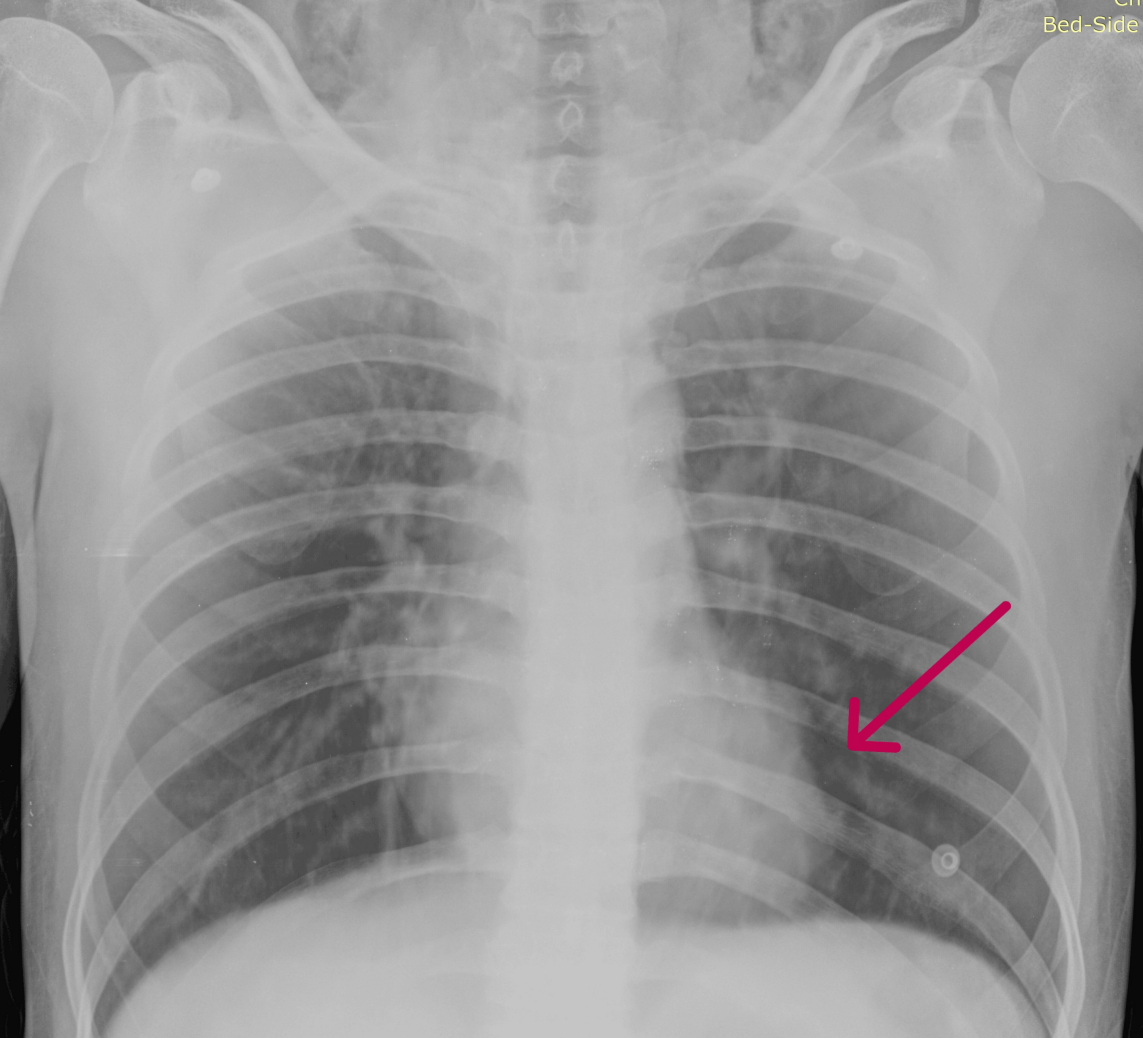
Chest X-ray showing air pocket in the mediastinum (taken July 24, 2022).
